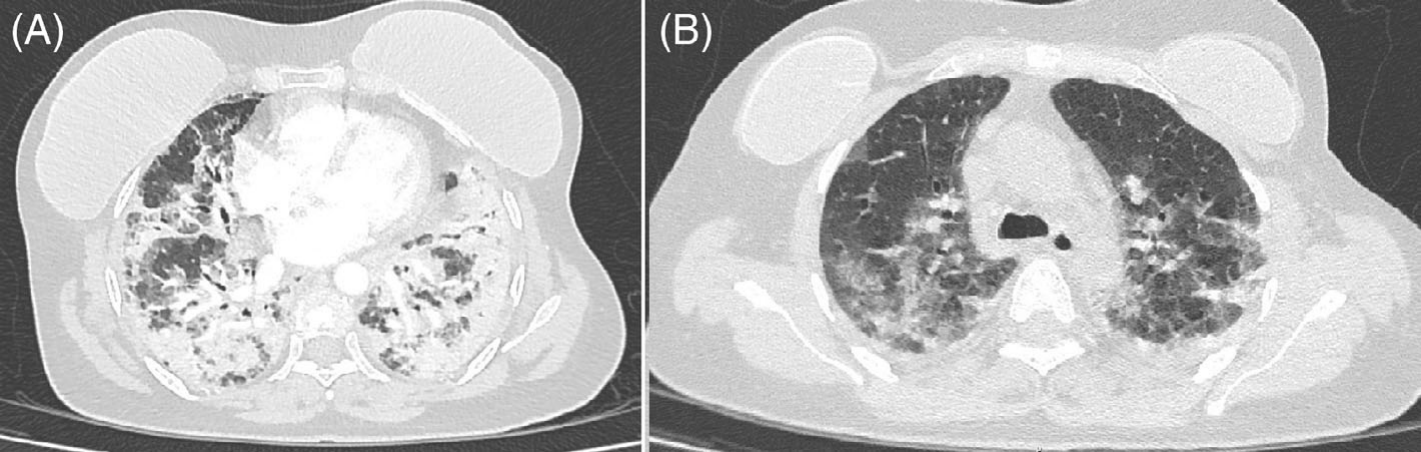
(A) A Computed tomography scan at presentation demonstrated confluent opacification with air‐ bronchograms consistent with pneumonic consolidation. (B) A higher slice showed patchy ground glass opacifications in both lung fields consistent with an interstitial process. Breast prostheses are visible on both slices
